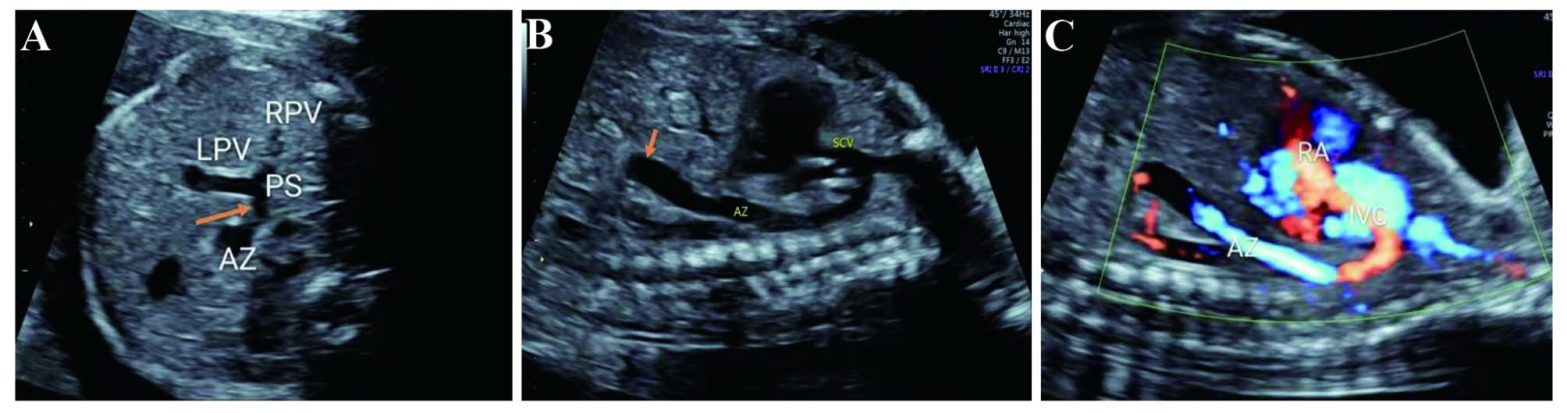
Ultrasonic images of another case of type IIIB UPSVS (extrahepatic portal-systemic shunts, EHPSS) (A-C) An abnormal blood vessel originated from the portal sinus, tortuously coursed to the left, posterior and upper, and merged into the azygos vein
